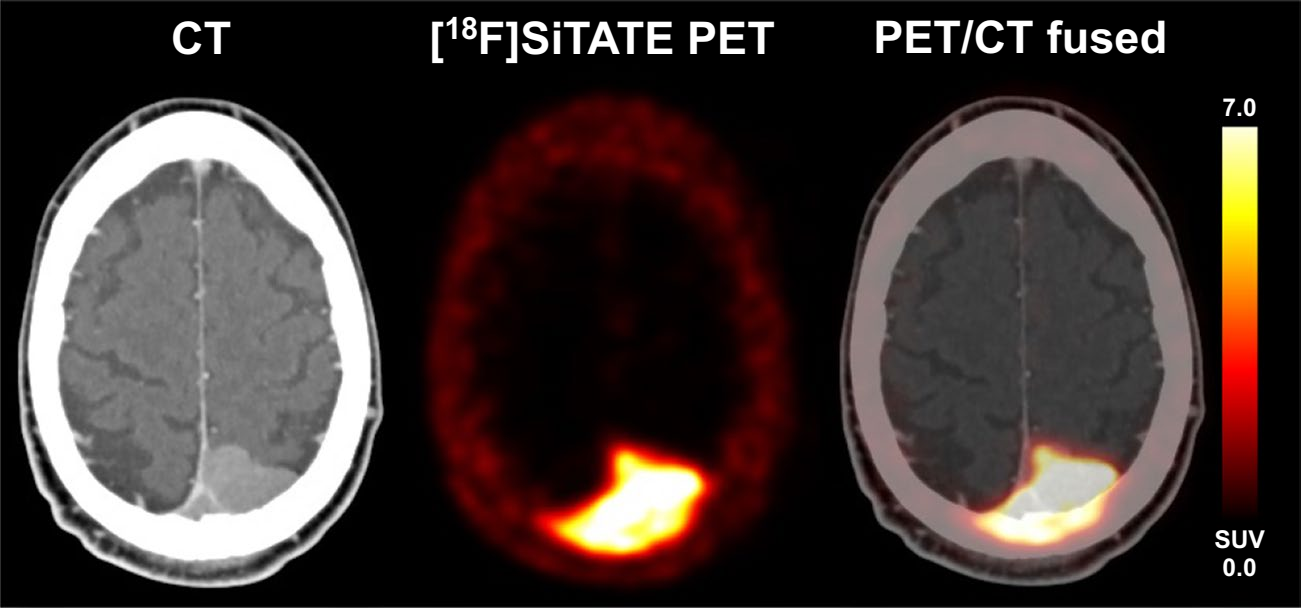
A case of a 51-year-old man with newly diagnosed occipital meningioma, which also showed infiltration of the super sagittal sinus with partial thrombosis and a trans-osseous extension within the occipital bone to the right side, which is easily detectable on [^18^F]SiTATE PET due to the strong SSTR expression (SUV_max_ 11.9). Resection revealed a CNS WHO grade 1 transitional meningioma
